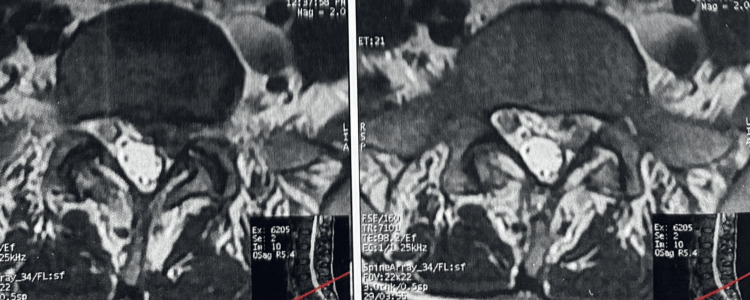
Postoperative axial lumbar MRI showing no evidence of recurrent disc herniation. The S1 nerve root appears enlarged and hyperintense, consistent with inflammatory radiculitis.
